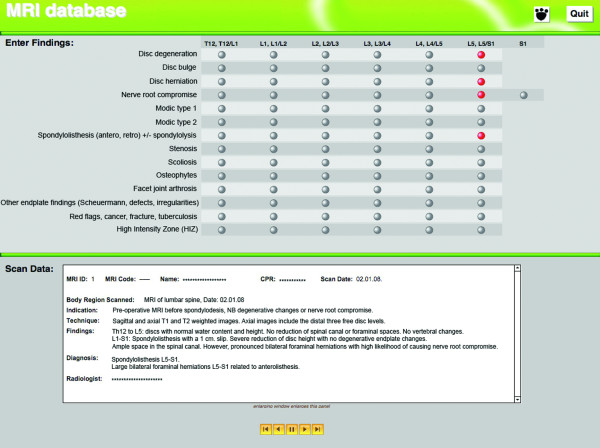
Screen shot of electronic coding matrix, consisting of 14 pathoanatomic categories for coding a narrative MRI report.
